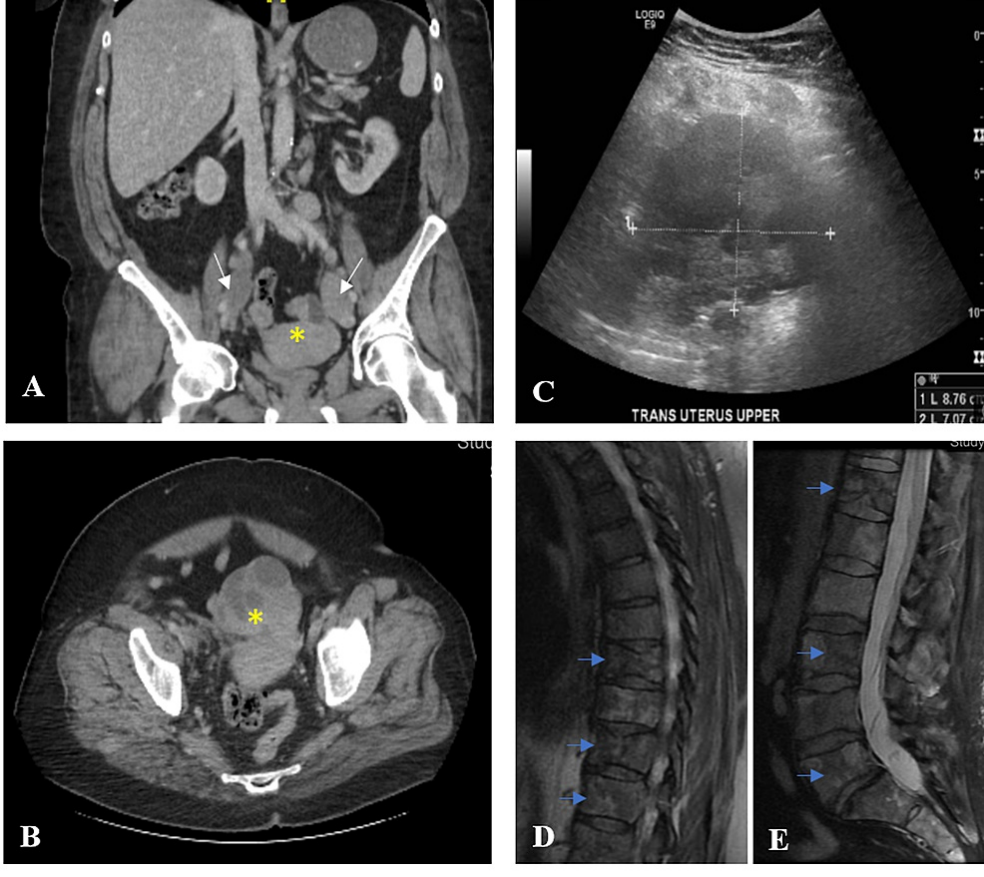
Representative CT images of pelvic mass and iliac adenopathy, and MRI showing metastasis to the spine Coronal (A) and transverse (B) views of abdomen/pelvic CT. White arrows indicate bilateral adenopathy; yellow asterisks indicate a large pelvic mass measuring 10 × 6 × 8 cm. (C) Pelvic mass, superior to the uterus, re-demonstrated on transvaginal pelvic ultrasound. MRI of the spine shows pathologic compression fractures with extensive and diffuse osseous metastatic disease (blue arrows) involving the thoracic (D) and lumbar (E) vertebrae. CT, computed tomography; MRI, magnetic resonance imaging.
